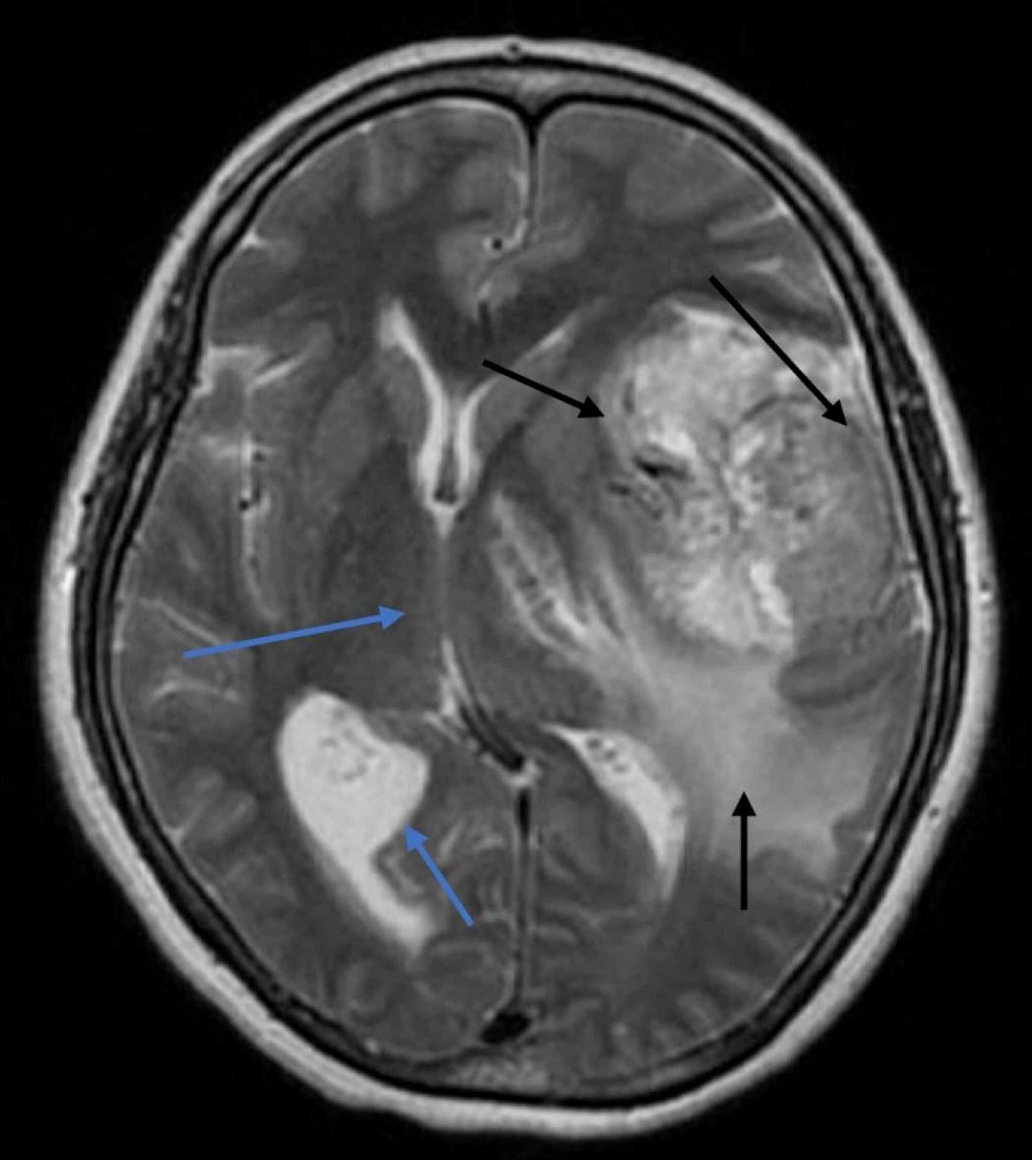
Axial FLAIR T2-weighted image of the patient’s hemisphere, showing hypointense to hyperintense irregular mass with parenchymal involvement and perilesional vasogenic edema (black arrows). The mass was causing midline shift, and mild dilation of the contralateral lateral ventricle was also identified (blue arrows). FLAIR: Fluid-attenuated inversion recovery.
